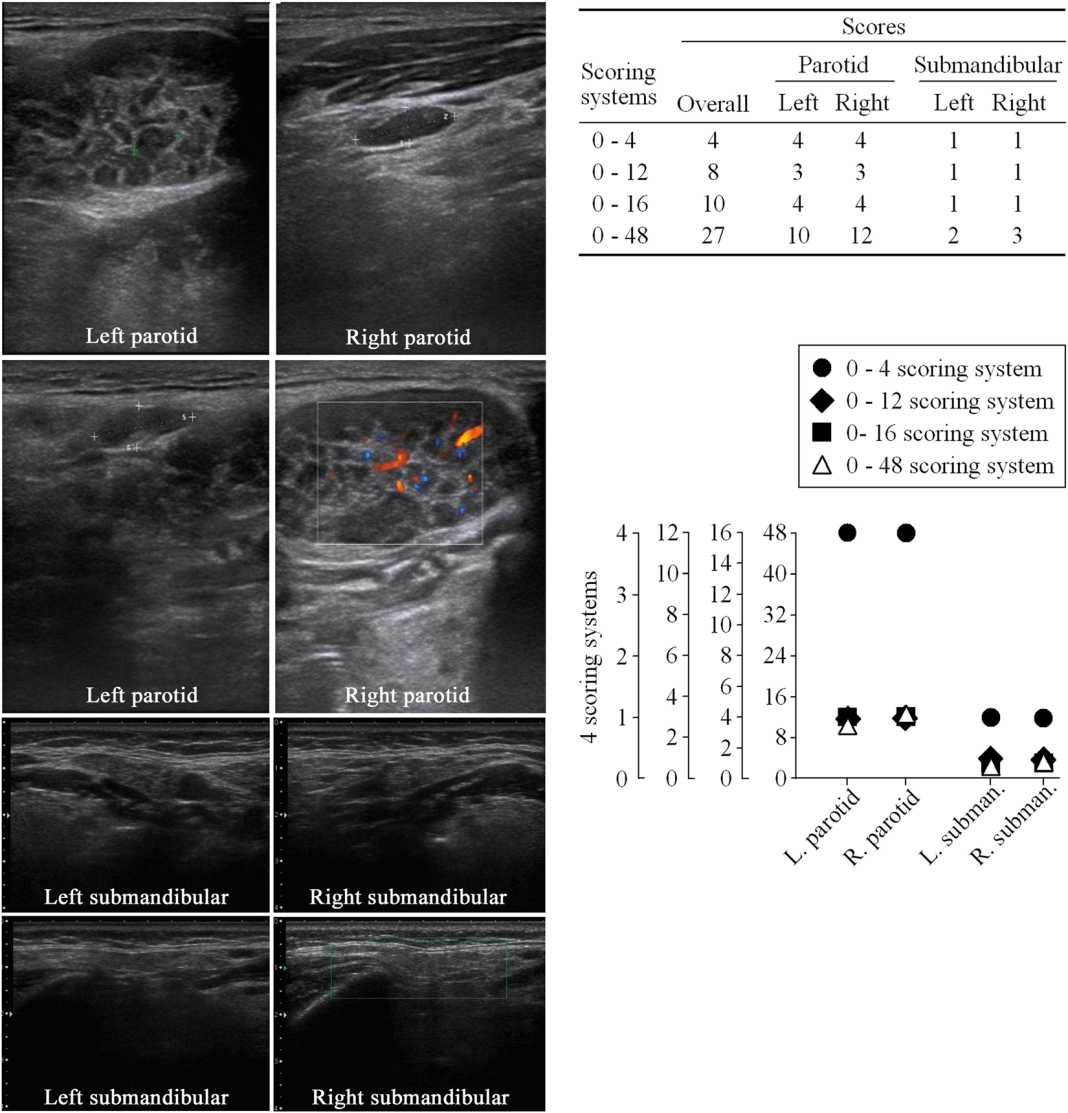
The ultrasound pictures of the parotid gland and submandibular gland from a patient (66 years old) diagnosed as Sjögren’s syndrome (left), the scores from different scoring systems (upper right), and direct comparison of different scoring systems (lower right).
